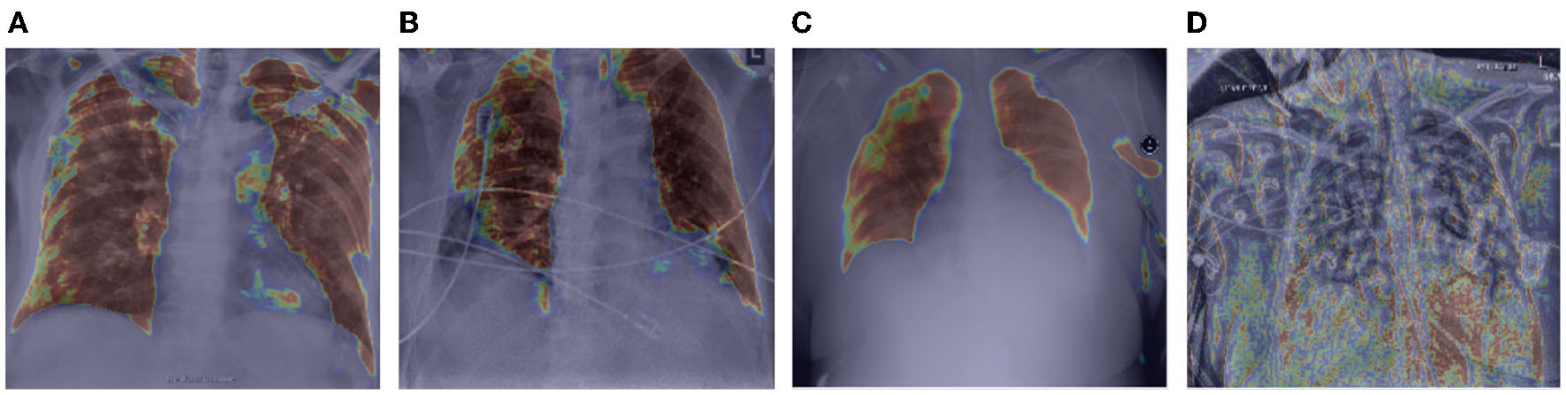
Example chest X-ray images (A–D) from the COVIDx CXR-2 benchmark dataset overlaid by the global attention output from the proposed MEDUSA network. The red regions indicate higher attention while blue regions indicate lower global attention.
